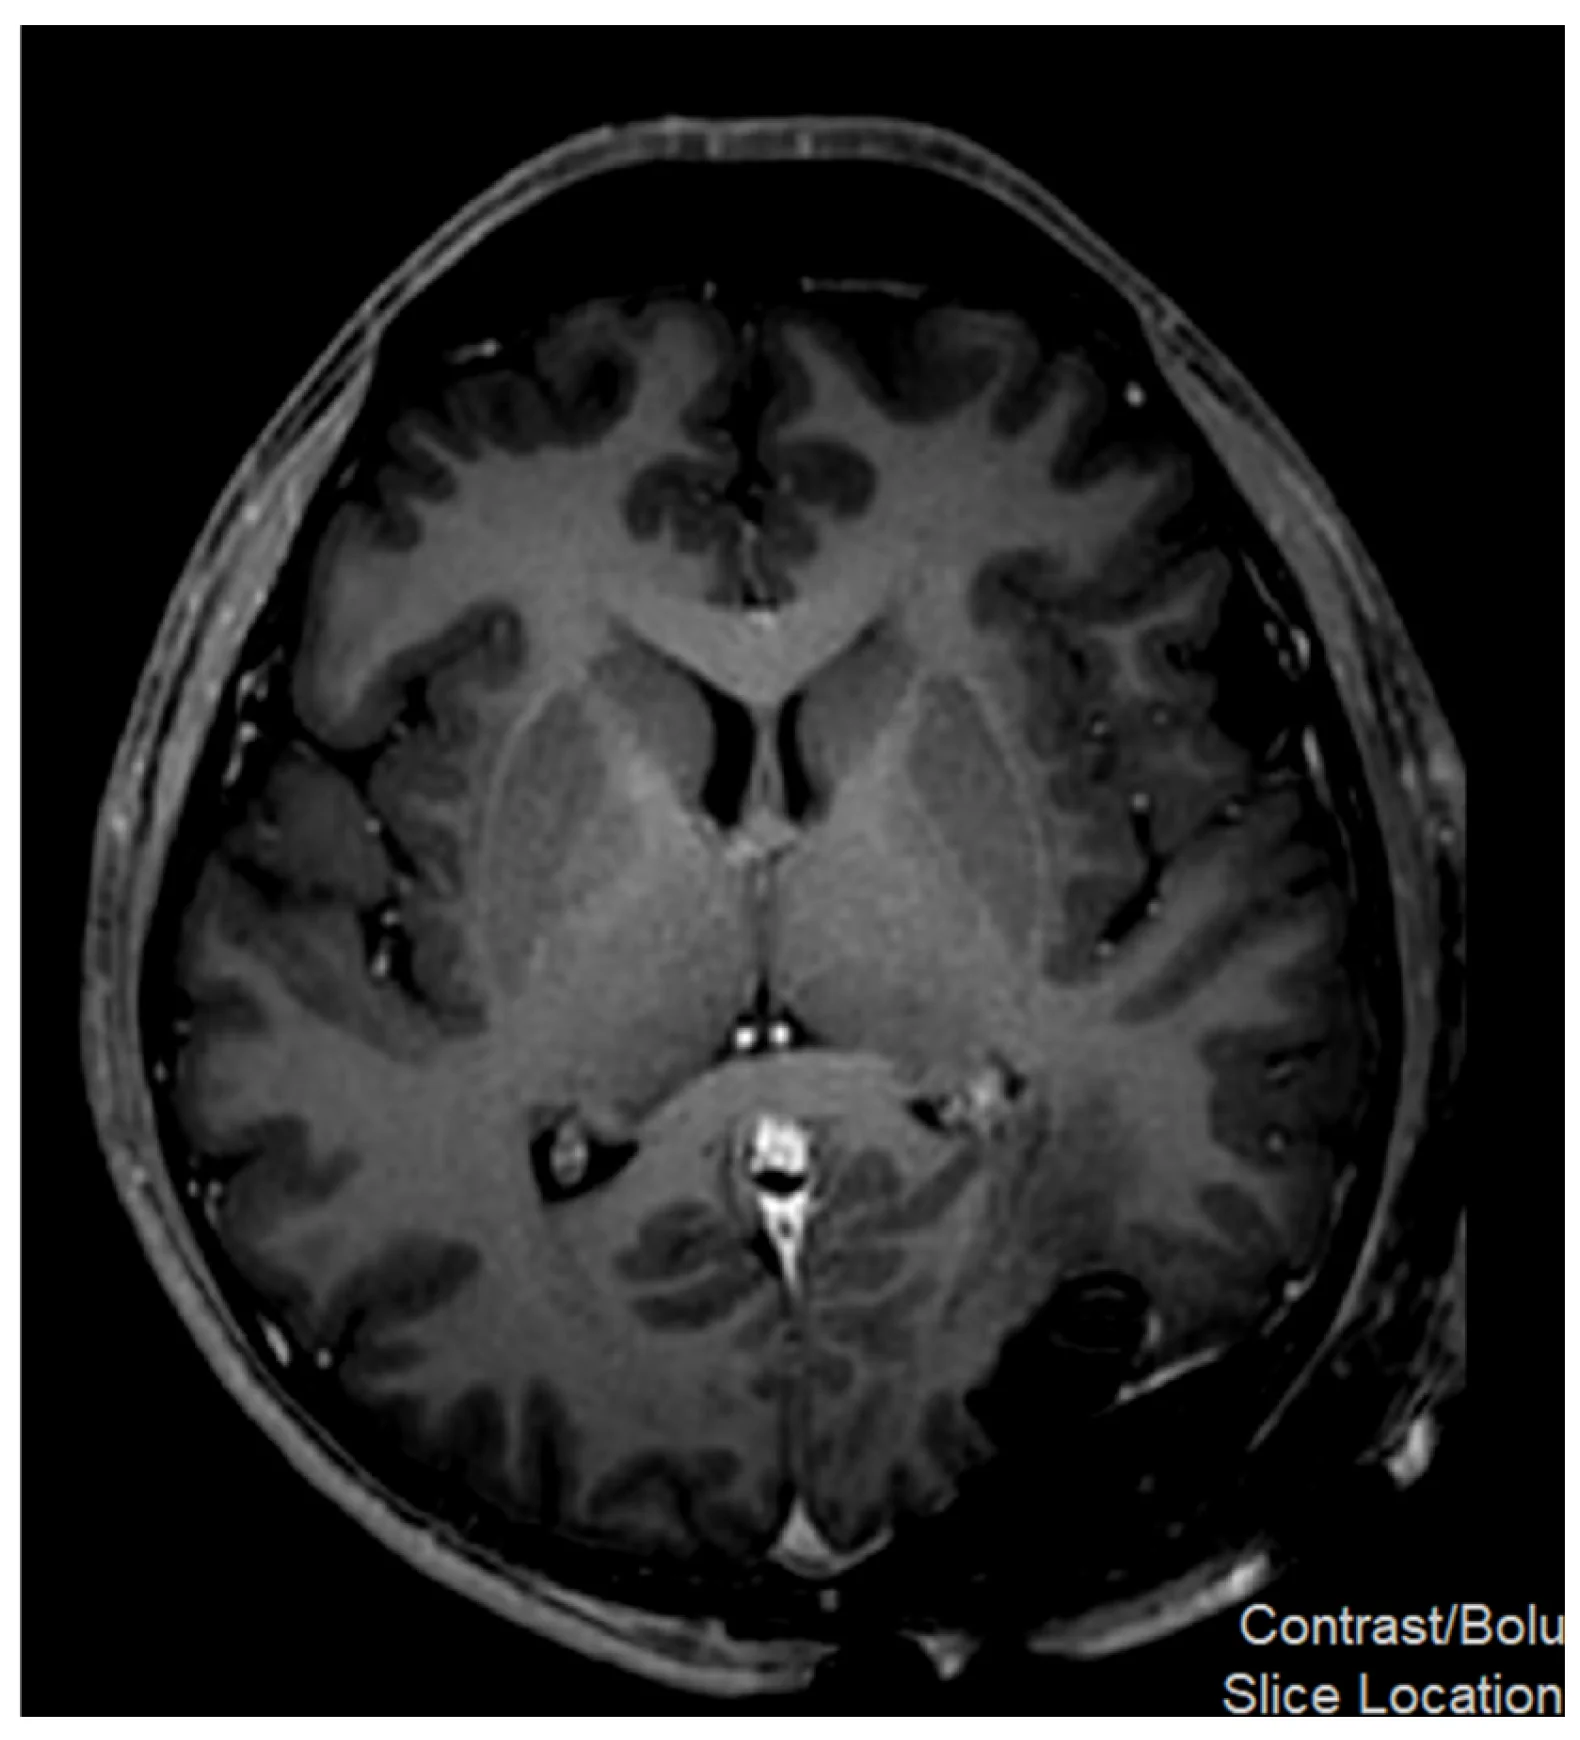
Postoperative brain MRI demonstrating complete excision of the left occipitoparietal lesion with no residual contrast enhancement.
